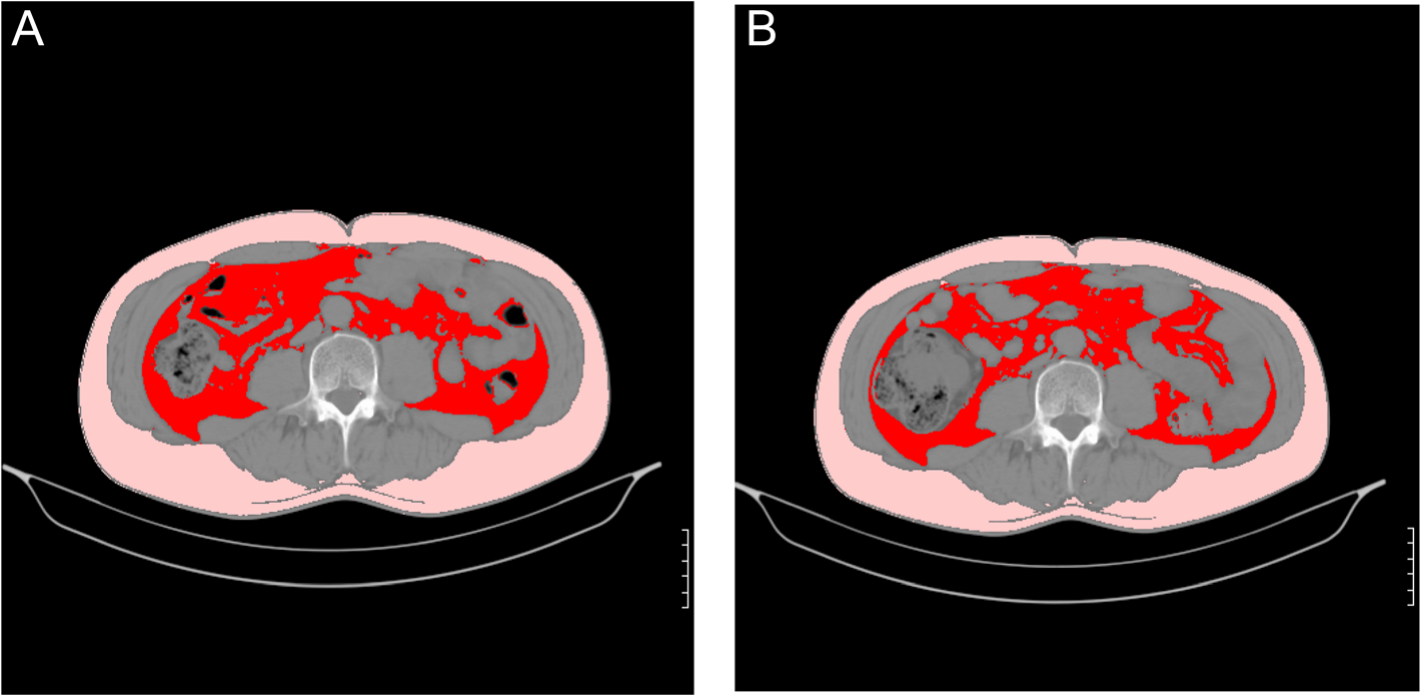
Representative CT scans demonstrating the change in visceral fat area (VFA) of the subjects fed lactic-fermented egg white (8 g as protein) for 8 weeks. Red: Visceral fat, Pink: Subcutaneous fat. a: Pre-intake (VF area = 107 cm^2^), b: Post-intake (VF area = 89 cm^2^)
